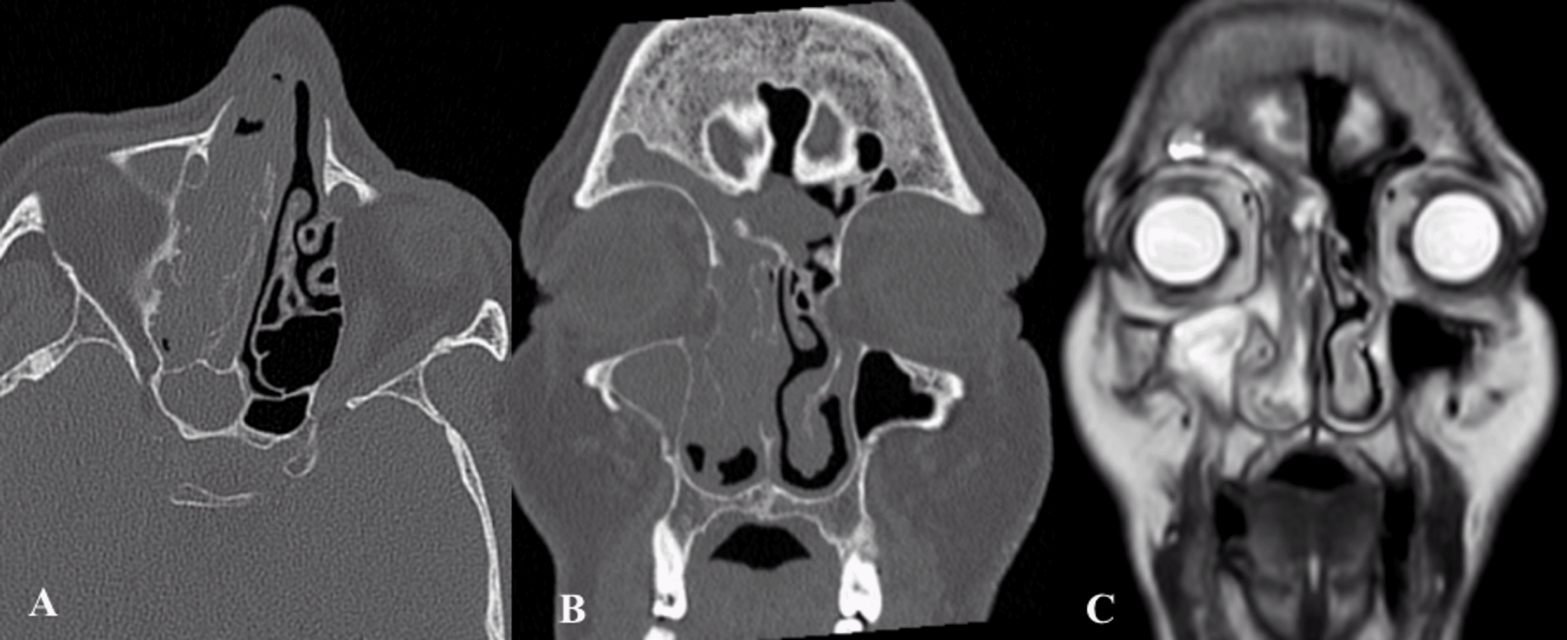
T4 IP Axial and coronal sections CT (A, B) and coronal section MRI (C) showing a T4 IP CT: computed tomography; IP: inverted papilloma; MRI: magnetic resonance imaging
